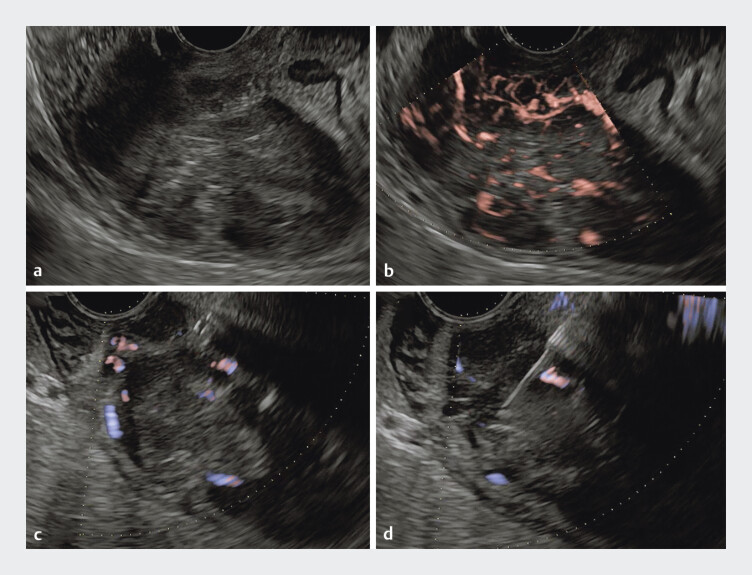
Ultrasonographic images of EUS-TA.
